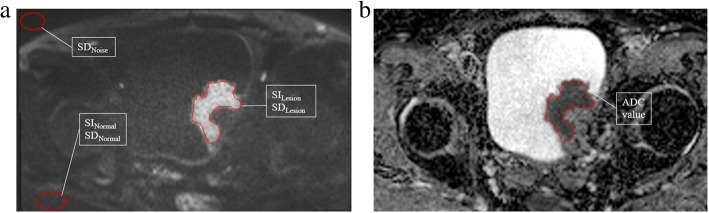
Representative images of outlined ROIs in bladder lesions. a DWI: b-value = 1000 s/mm^2^. ROIs were delineated to determine the signal intensity and standard deviation of the lesion and normal reference tissues, as was the standard deviation of the noise level. b ROI outlined for estimating the ADC value of the bladder lesion
